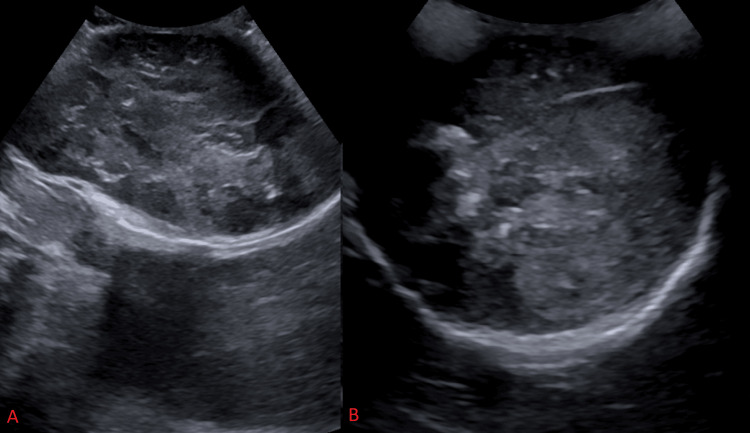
Scrotal ultrasound with sagittal (A) and transverse (B) views demonstrating an 11.3 x 6.8 x 8cm heterogenous mass in the left scrotal sac.
